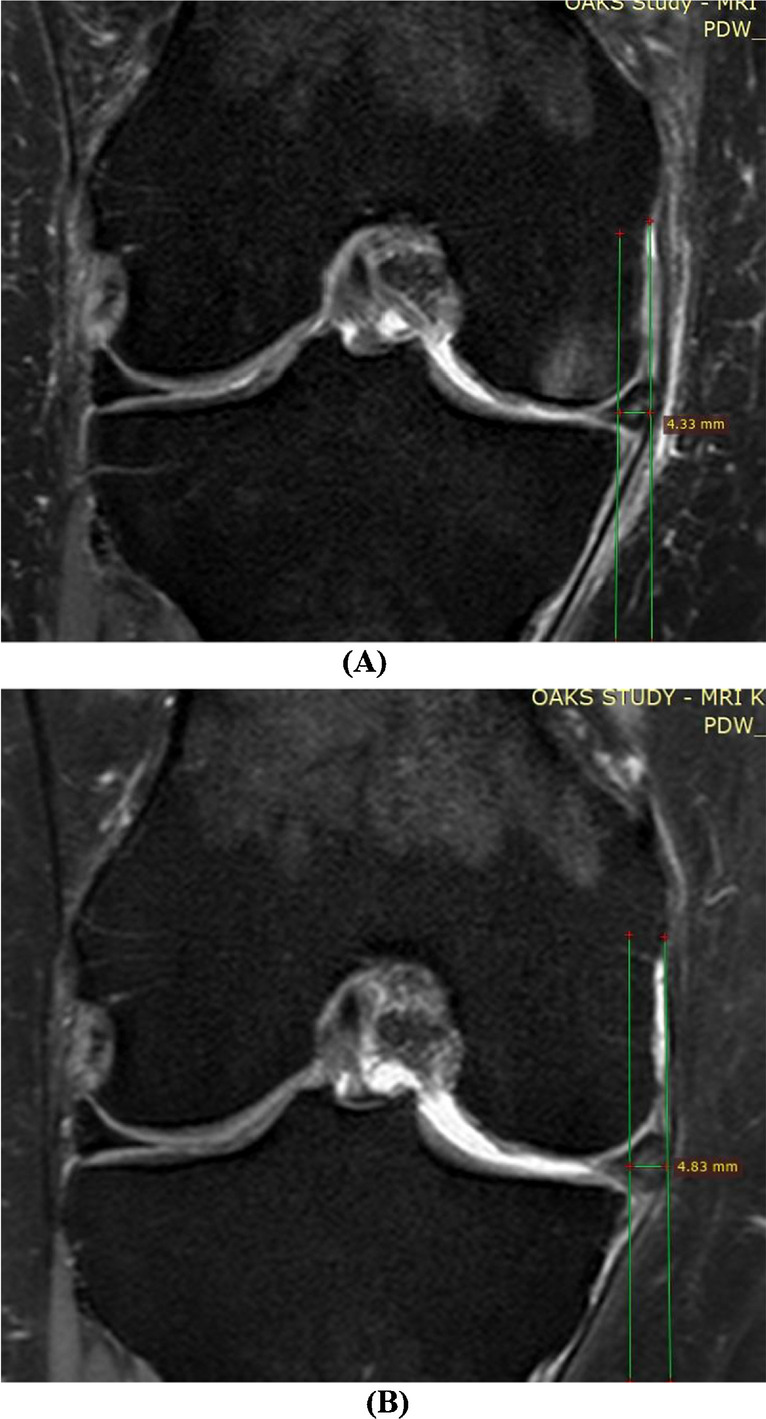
Measurement of medial meniscal extrusion. A Baseline MRI; B follow-up MRI of the same participant. Medial meniscal extrusion was measured on the coronal plane at the slice with the largest area of the medial tibial spine with the steps: (1) drop a perpendicular line at the point of medial tibial plateau transitioning from horizontal to vertical; (2) drop another perpendicular line intersecting the outermost edge of the medial meniscus; (3) measure meniscal extrusion as the distance between the two perpendicular lines
